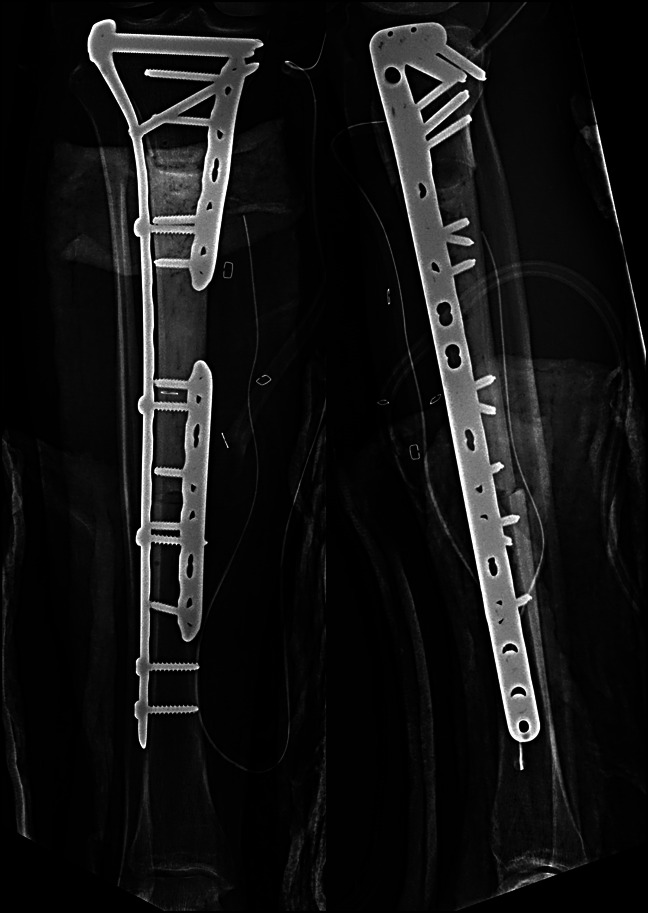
Radiograph of a 28-year-old woman demonstrating hemispherical reaming at proximal tibia metadiaphysis and mid-diaphysis graft-host interface with low-contact dynamic compression plate fixation (case 2: AP and lateral radiographs after intercalary allograft reconstruction on postoperative day zero).
